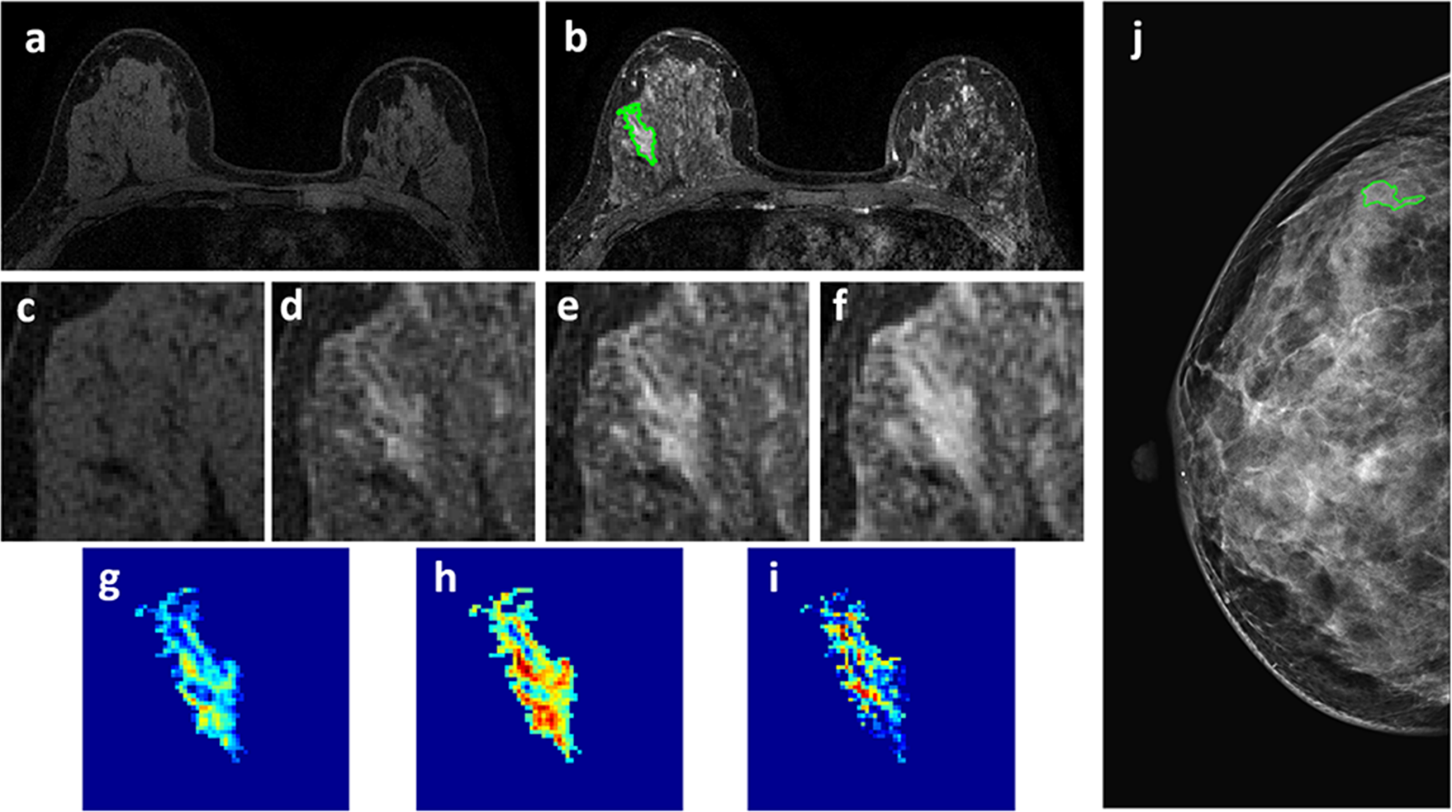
A 46-year-old patient with a 2.7 x 1.3 cm benign adenosis. This is a young woman with extremely dense breasts showing substantial parenchymal enhancements. The lesion shows a persistent DCE-MRI pattern and determined as BI-RADS 4A on MRI. (A) F1 Pre-contrast image. (B) F2 post-contrast image. (C–I) Magnified images to demonstrate the margin and internal enhancements within the lesion. (C) F1 pre-contrast, (D) F2 post-contrast, (E) F3 post-contrast, (F) The last F6 post-contrast image. (G) The wash-in signal enhancement map F2-F1, (H) The F3-F1 signal enhancement map, (I) The wash-out F6-F3 map. (J) The lesion is not seen on mammography, determined as BI-RADS 2, and an area is outlined with the guidance of MRI. The probability predicted by MRI, mammography, and combined radiomics models were: 0.3, 0.41, 0.11, respectively, true negative.
